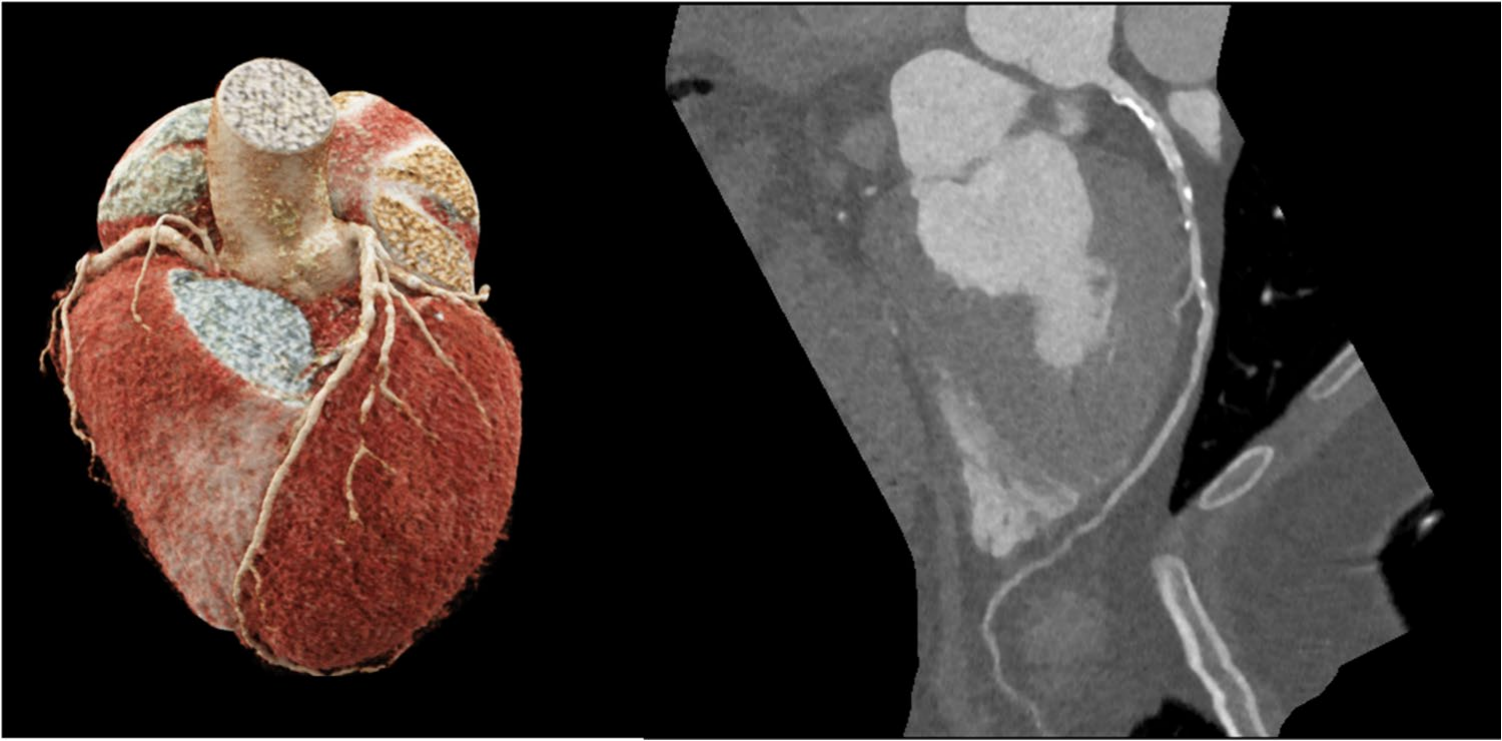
CT coronary angiography. The image on the left shows a 3D model of the coronary arteries. The right image displays a radial series of the left anterior descending artery of the same patient with calcified and non-calcified plaque in the proximal segment
